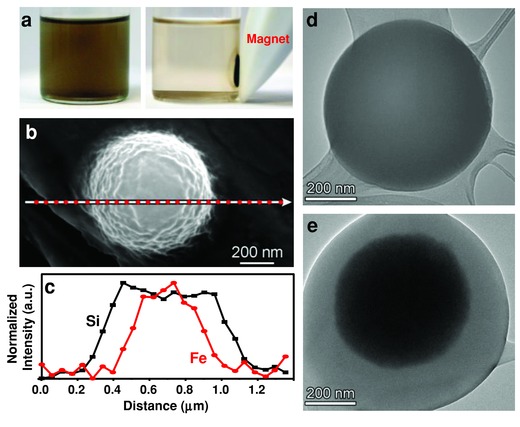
Magnetic core‐shell polydimethylsiloxane (PDMS) microspheres prepared using ultrasonic spray pyrolysis. a) Image of magnetic PDMS microspheres showing (left) microspheres dispersed in hexanes and (right) microspheres pulled from solution using a magnet. b) SEM of magnetic PDMS microsphere on copper foil showing path of energy‐dispersive X‐ray spectral (EDS) line scan. c) EDS line scan showing Si and Fe concentrations along the path line shown in (b). d) TEM of PDMS microsphere (prepared without Fe_3_O_4_) showing uniform density and composition throughout the sphere. e) TEM of magnetic microsphere showing core‐shell structure.
